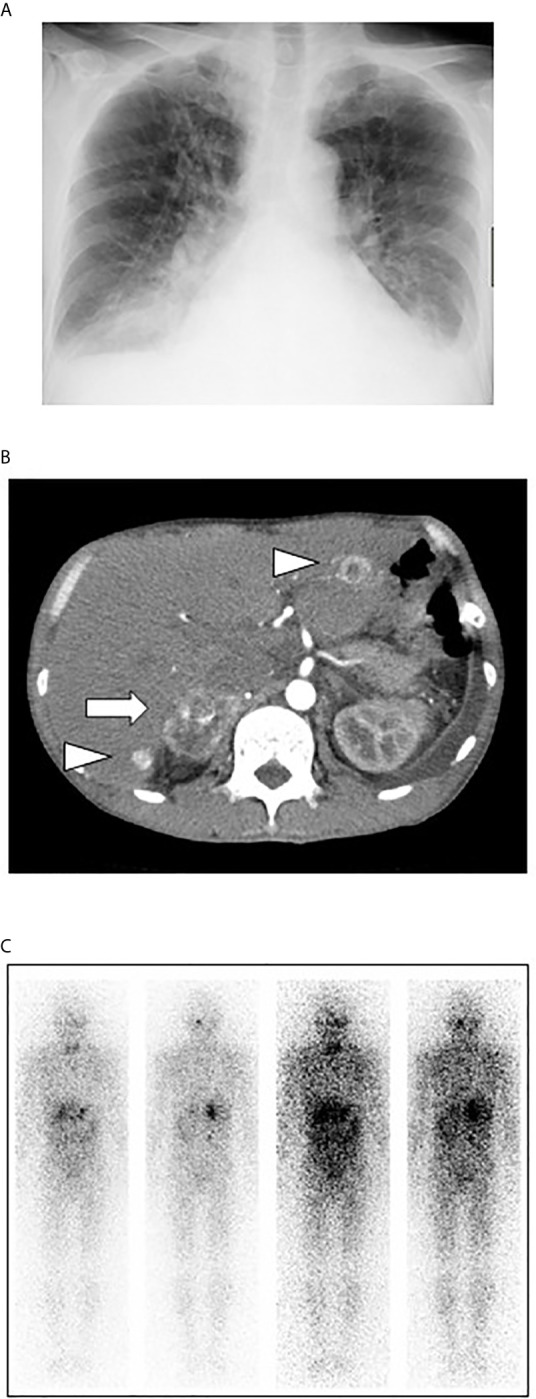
(A) In chest X-ray, permeability was reduced in bilateral lower lung field and butterfly shadow was observed. Cardio-thoracic ratio (CTR) was 59.8% and both costophrenic angles (CPA) were blunt. (B) Abdominal contrast-enhanced computed tomography revealed a 50-mm hyper-vascularized tumor with calcification in the right adrenal gland and multiple hyper-vascularized tumors in the liver. The right adrenal gland showed round shape and a mixture of high- and low-density area. In addition, since there was thin adipose tissue between the right adrenal gland and the kidney and inferior vena cava, there seemed to be no infiltration into surrounding organs. (C) In ^131^I MIBG scintigraphy, there was high accumulation in the right adrenal gland and multiple accumulation in the liver, and there was small hot spot in the sternum and right rib.
